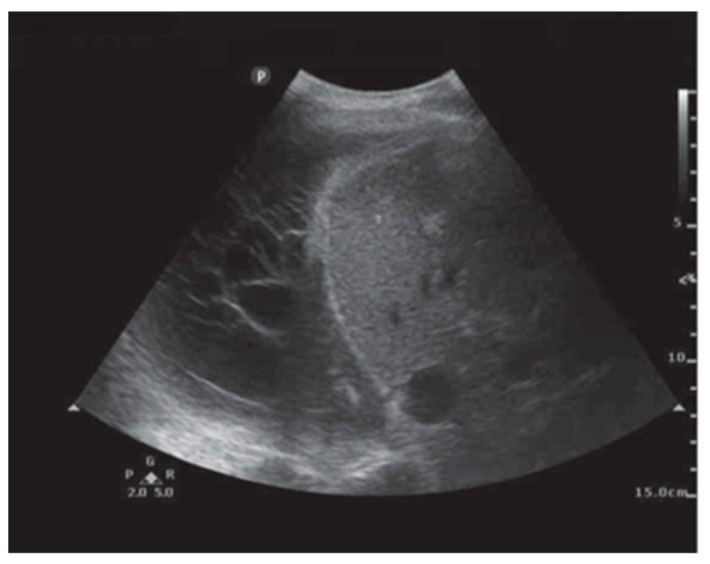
Pleural ultrasound revealing septations. Reproduced from Hassan et al., 2020 [28]. Shared under the terms of the Creative Commons Attribution Non-Commercial Licence 4.0. See: https://creativecommons.org/licenses/by-nc/4.0/.
